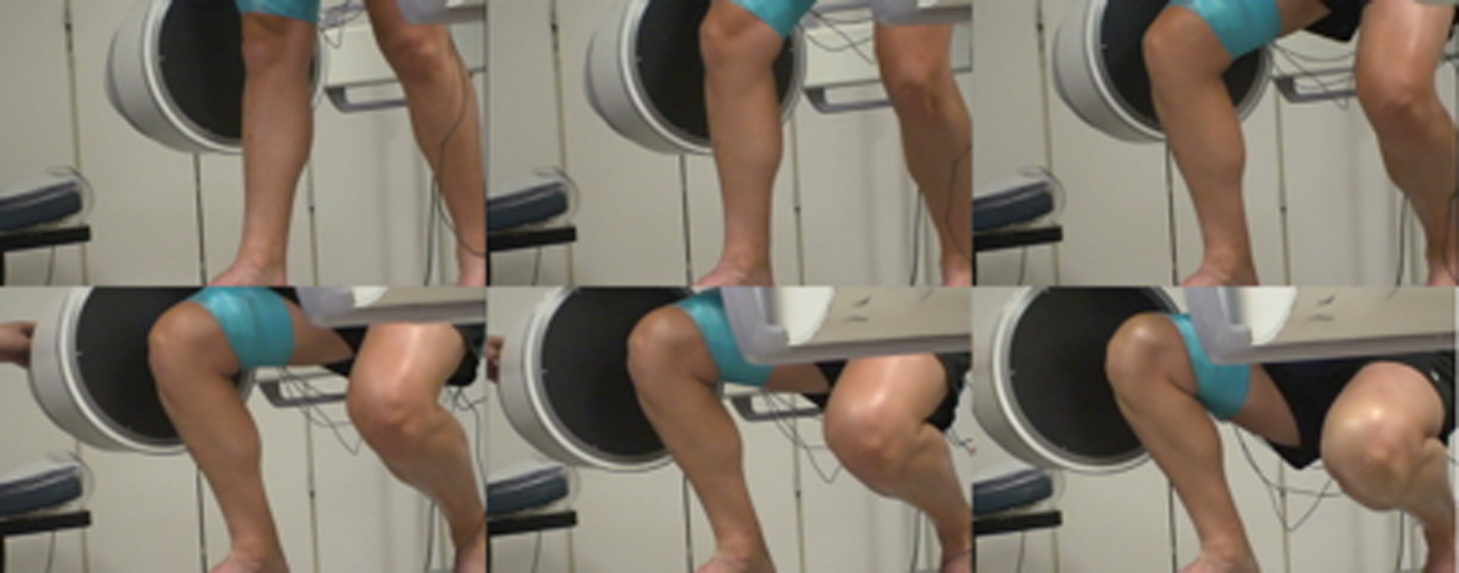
Monoplane fluoroscopic x-ray system for DKB.
